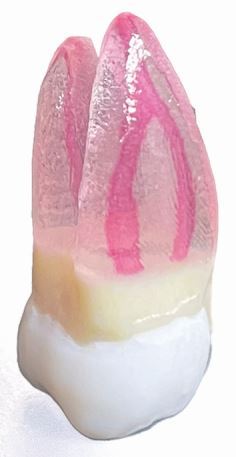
Full procedural stages including: Tooth, root and canal assessment Access Canal identification Biomechanical preparation (De)obturation Post preparation Restoration Surgical endodontics (with advanced model development)	Fully anatomical Good visualisation of procedural errors and obturations without radiographs (if translucent roots) Standardised design for each student and cohort which aids: Staff calibration for feedback Objective intra-year and inter-year comparison of performance and teaching practices Enables in-house production	Expensive if purchased High initial equipment costs and time-consuming if designed and produced in house Softer tactile feedback cf. enamel and dentine Artificial dentine colour changes for canal identification Acrylic/resin smear layer that can predispose to blockages, fractures and file separations Small canal diameters may be below available printer resolution (print-technology dependent) Difficulty removing support materials within root canal system
